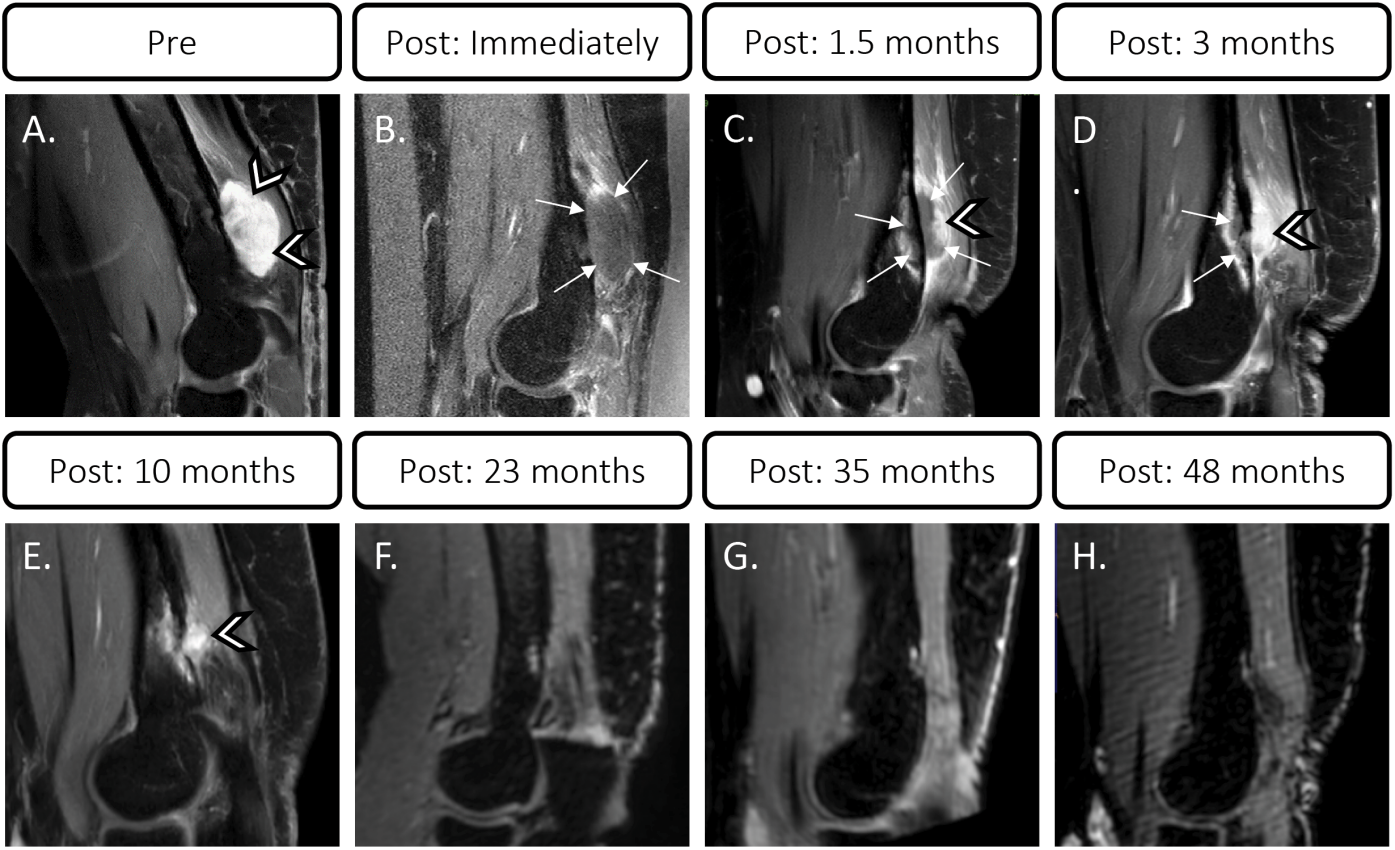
Contrast-enhanced T1-weighted MR images of desmoid tumor before and after MR-HIFU treatment. (A) Hyperintense desmoid tumor at dorsal humerus (arrow heads) before MR-HIFU. (B) Non-enhancing ablation zone immediately after treatment (arrows) with NPV ratio of 95%. (C, D) Tumor (arrow heads) at follow-up showing NPV (arrows) with hyperemic rim at 1.5 and 3 months after treatment. (E) Tumor at 10 months post MR-HIFU. (F) Complete resolution of desmoid tumor at 23 months post MR-HIFU. No tumor recurrence at 35 (G) and 48 months (H) after treatment.
